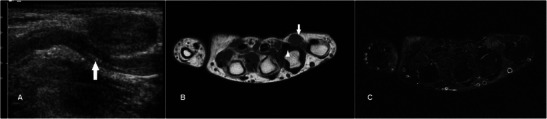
Inflammatory pseudotumour in a 50-year-old female presenting with a painless mobile lump over the medial aspect of the palm for a few months. (a) Ultrasound shows a solid heterogeneous lesion in the palm over the fourth web space close to the flexor tendon of the ring finger (arrow). (b) T1w sequence shows a lobulated subcutaneous nodule of intermediate signal (arrow) close to but separate from the flexor tendon of ring finger (arrowhead). (d) The lesion is only minimally hyperintense on T2w-FS sequence. No intravenous contrast was given because of renal impairment
